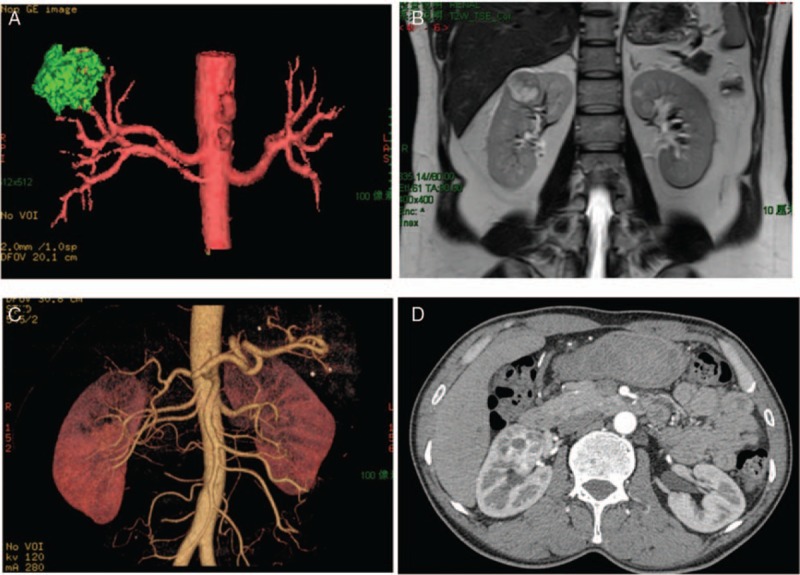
Showed the similar ability of detecting aberrance renal arteries in noncontrast-enhanced magnetic resonance angiography (A) and (B) and computed tomographic angiography (C) and (D).
